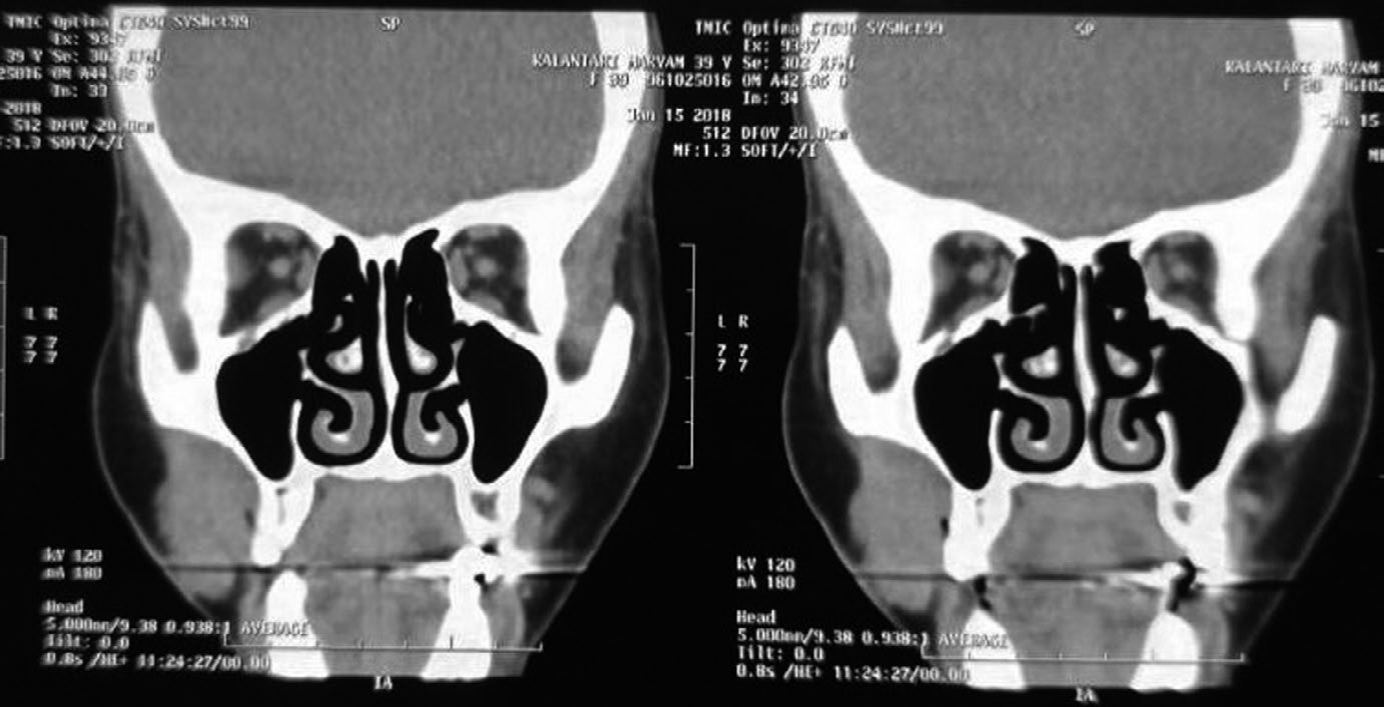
CT scan view of the lesion demonstrates ill‐defined mass of right buccal area with invasion to the surrounding structures of the skin and zygomatic bone
